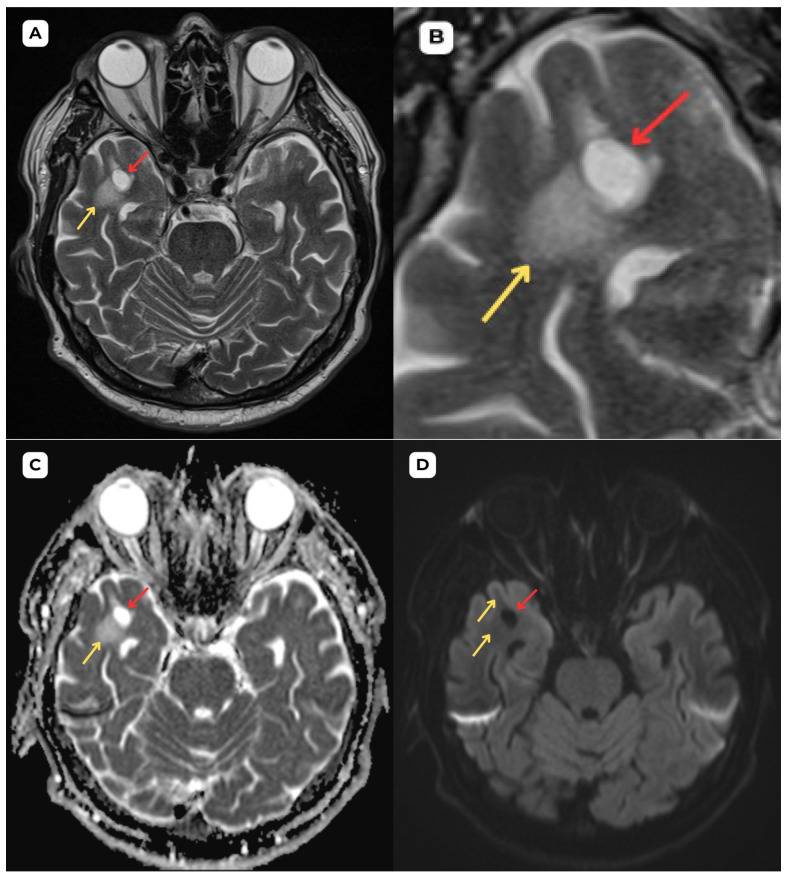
First patient: One-year follow-up brain MRI of a 63-year-old male. (A) Axial T2-weighted sequence and (B) axial T2-weighted sequence zoomed in. The one-year follow-up shows no changes in the cystic lesion or surrounding structures, supporting the diagnosis of a benign process, consistent with an opercular perivascular space (type IV). The surrounding edema seen previously has remained stable, with no increase in size or signal intensity, further suggesting the lesion’s non-progressive, benign nature. One-year follow-up shows no changes in (C) high ADC map values and (D) no diffusion restriction on DWI B1000. Red arrow—opercular (type IV) perivascular space; yellow arrow—surrounding edema.
